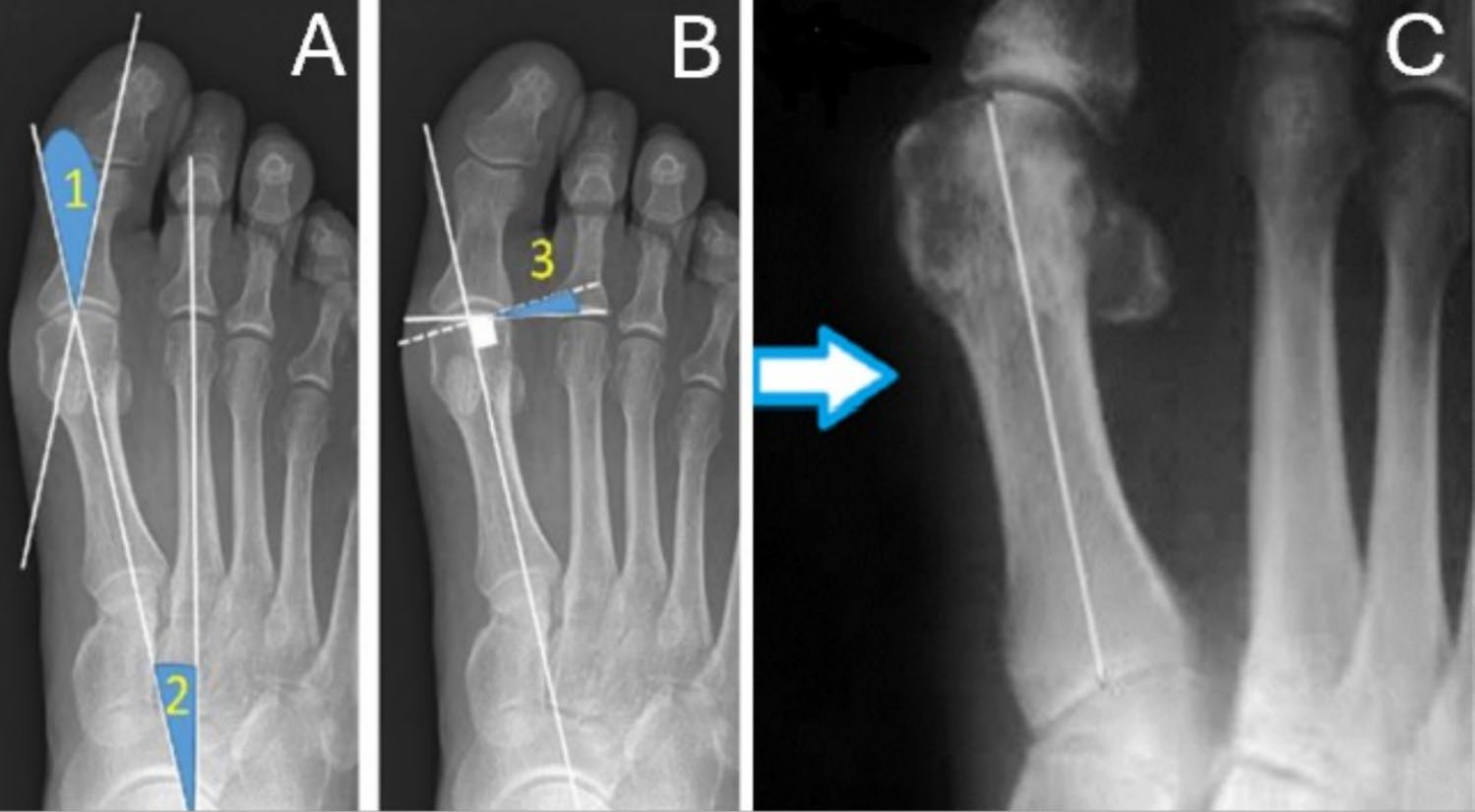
Measurements performed on standard dorsoplantar weightbearing radiographs. A 1: hallux valgus angle; 2: intermetatarsal angle; B 3: distal metatarsal articular angle; C tibial sesamoid position (arrow indicating the tibial sesamoid)
